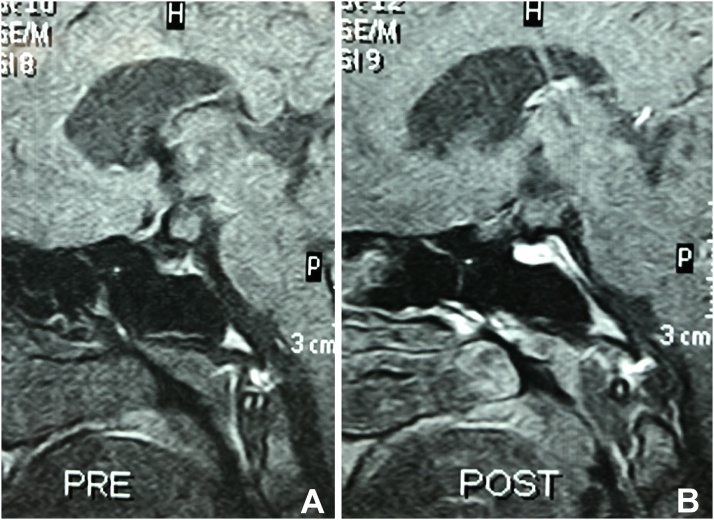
Post-operative MRI of the brain showed an evidence of poorly enhancing lesion in the hypothalamic region suggesting recurrence.
